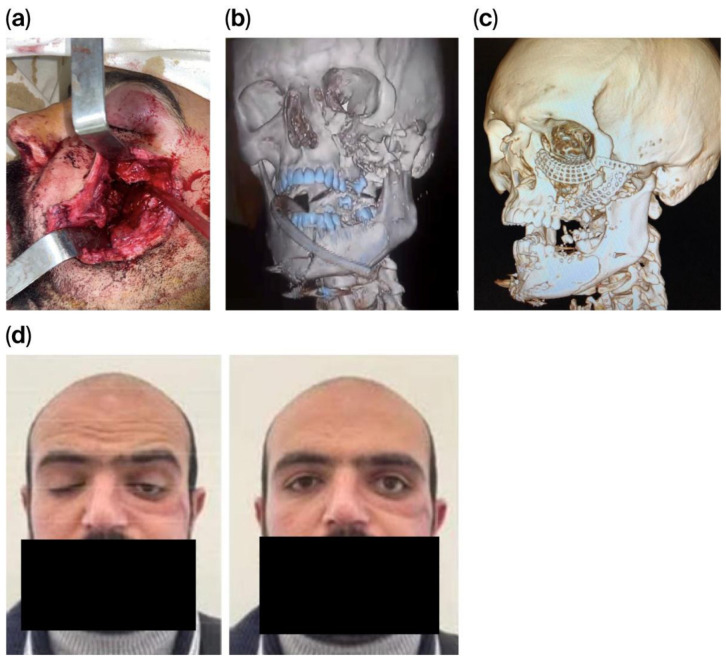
Drone-blast open midfacial and mandible injury: (a) 3D CT scan demonstrates left-site severely comminuted multifragmental midface (zygomatico-orbital, NOE, and zygomatico-maxillary complex) and mandible fractures; (b) early definitive wound access reconstruction osteosynthesis using titanium mesh and plates; (c) postoperative 3D CT scan; (d) long-term (5-year follow-up) postoperative result demonstrating soft tissue thinning over titanium mesh and plates in the left zygomatic region; lower left eyelid deficit malocclusion because of second upper left molar disposition.
